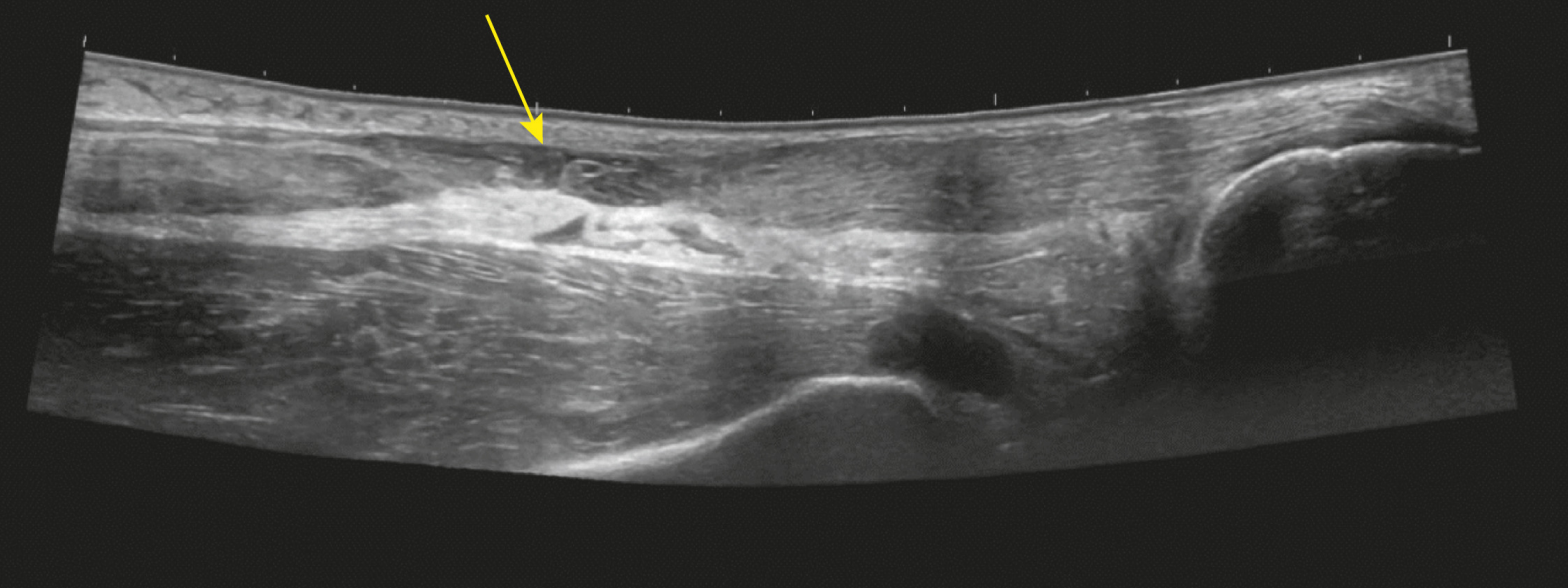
Hospital ultrasound sagittal view of the Achilles rupture.
